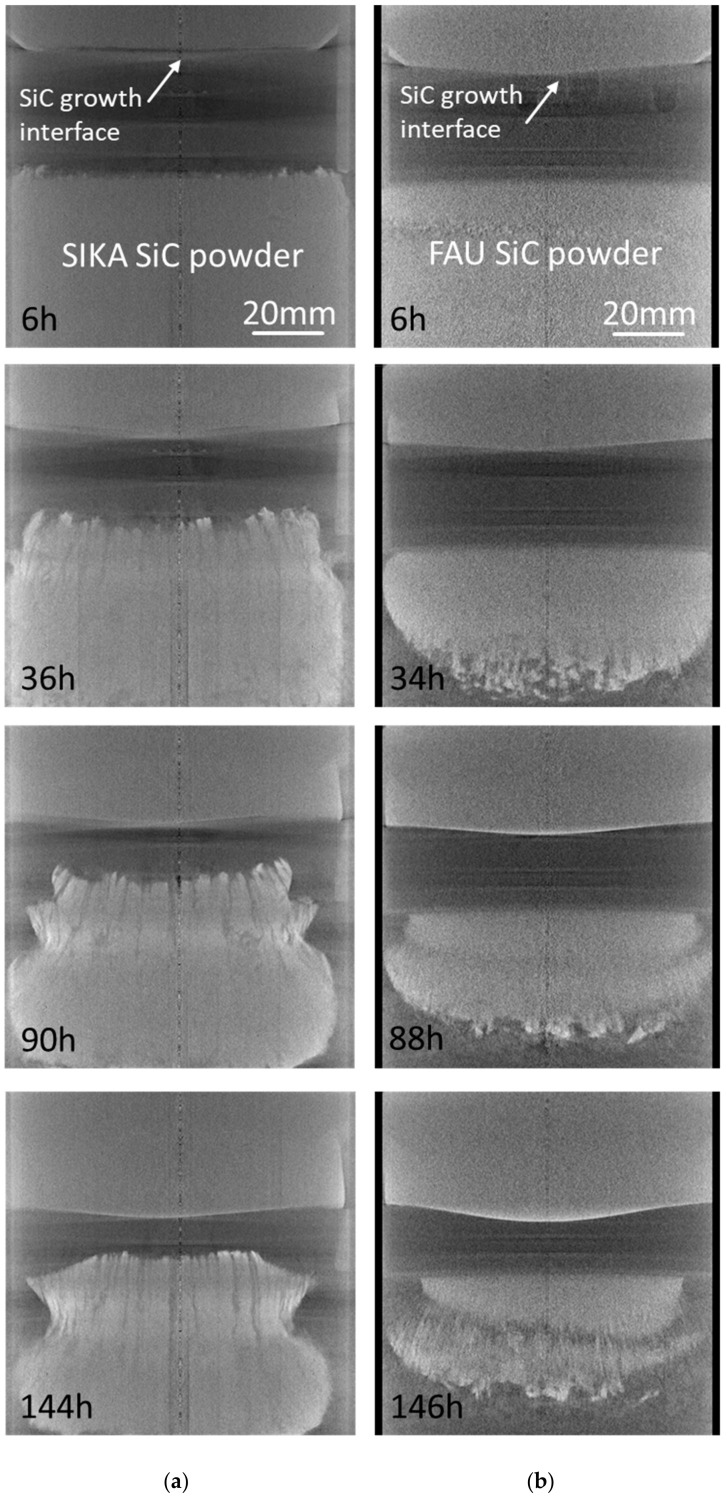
Series of 2D projections of the 3D in situ X-ray-based computed tomography visualization of the growth process of the 75 mm PVT growth process: (a) SiC powder from SIKA and (b) reference SiC powder from FAU.
